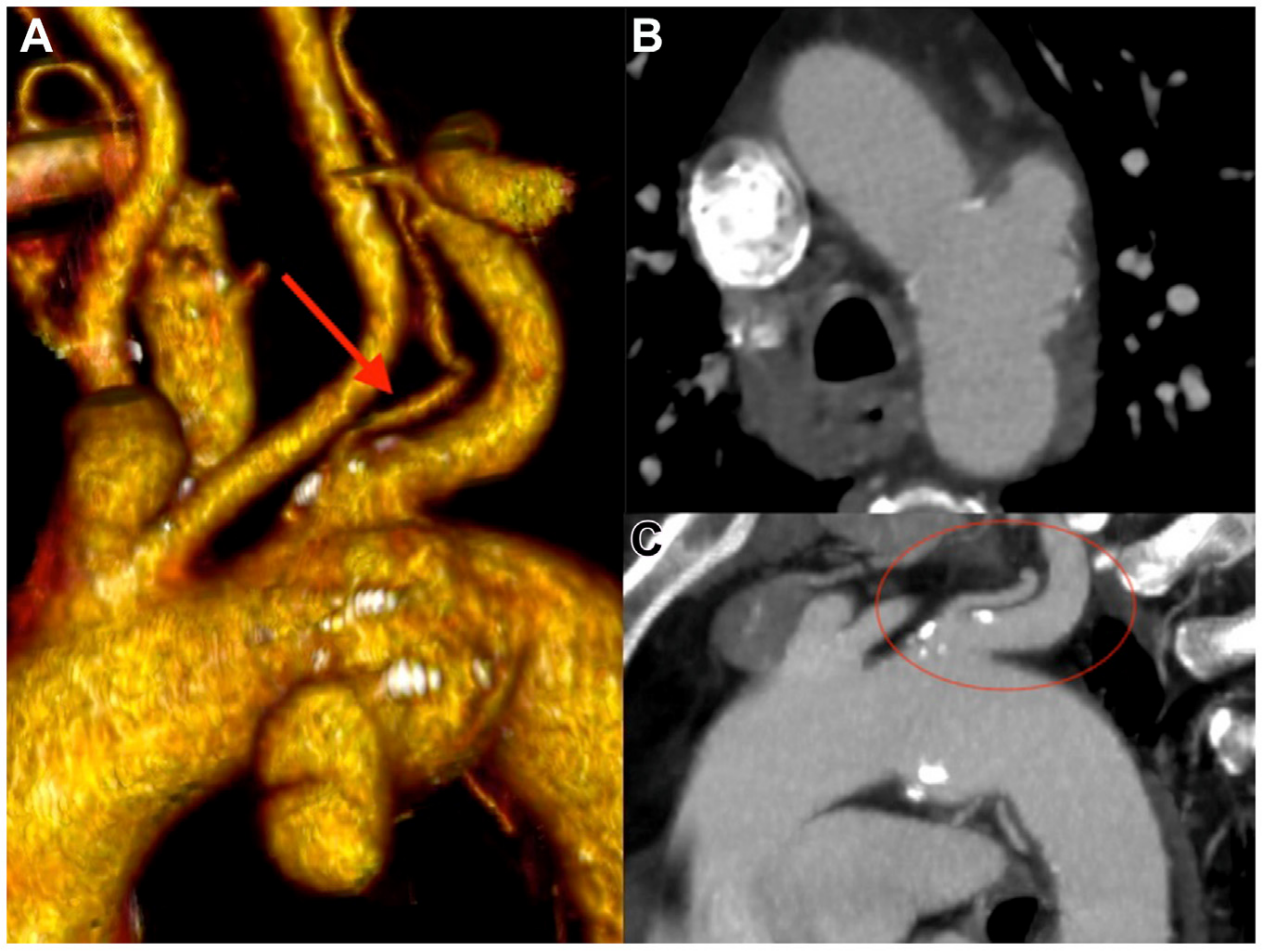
(A-C) Computed tomography angiography (CTA) showing the zone 2 penetrating aortic ulcer of the arch. The left vertebral artery (LVA) and its origin are highlighted (A, red arrow; C, red circle).
